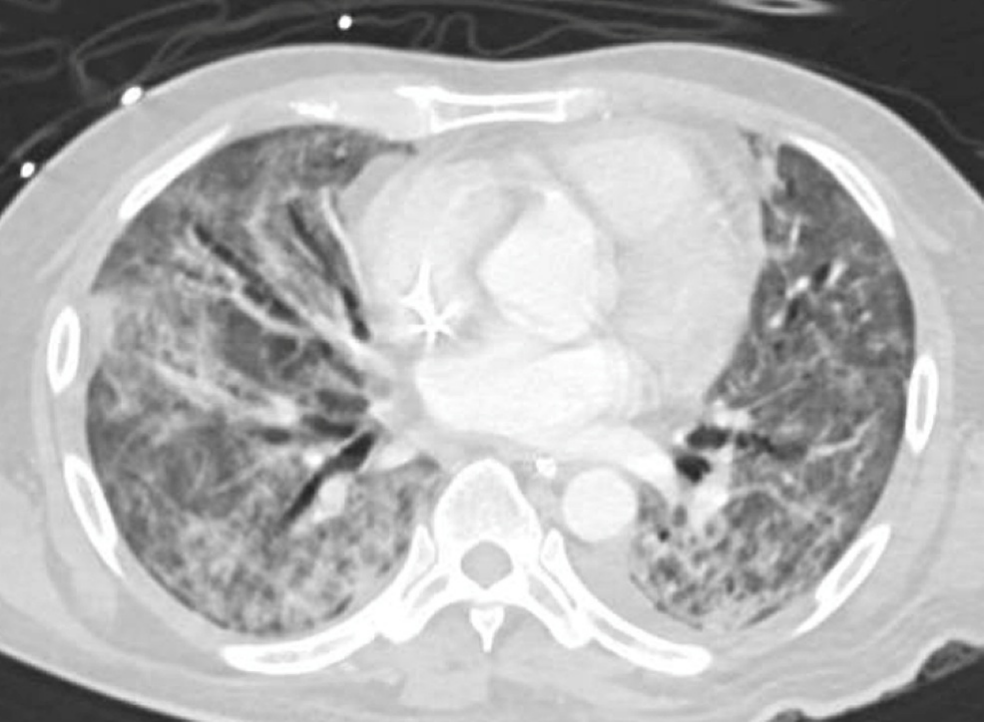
CT-chest showing bilateral consolidation and ground-glass opacities with air bronchogram and evidence of bronchiectasis more on the right side
